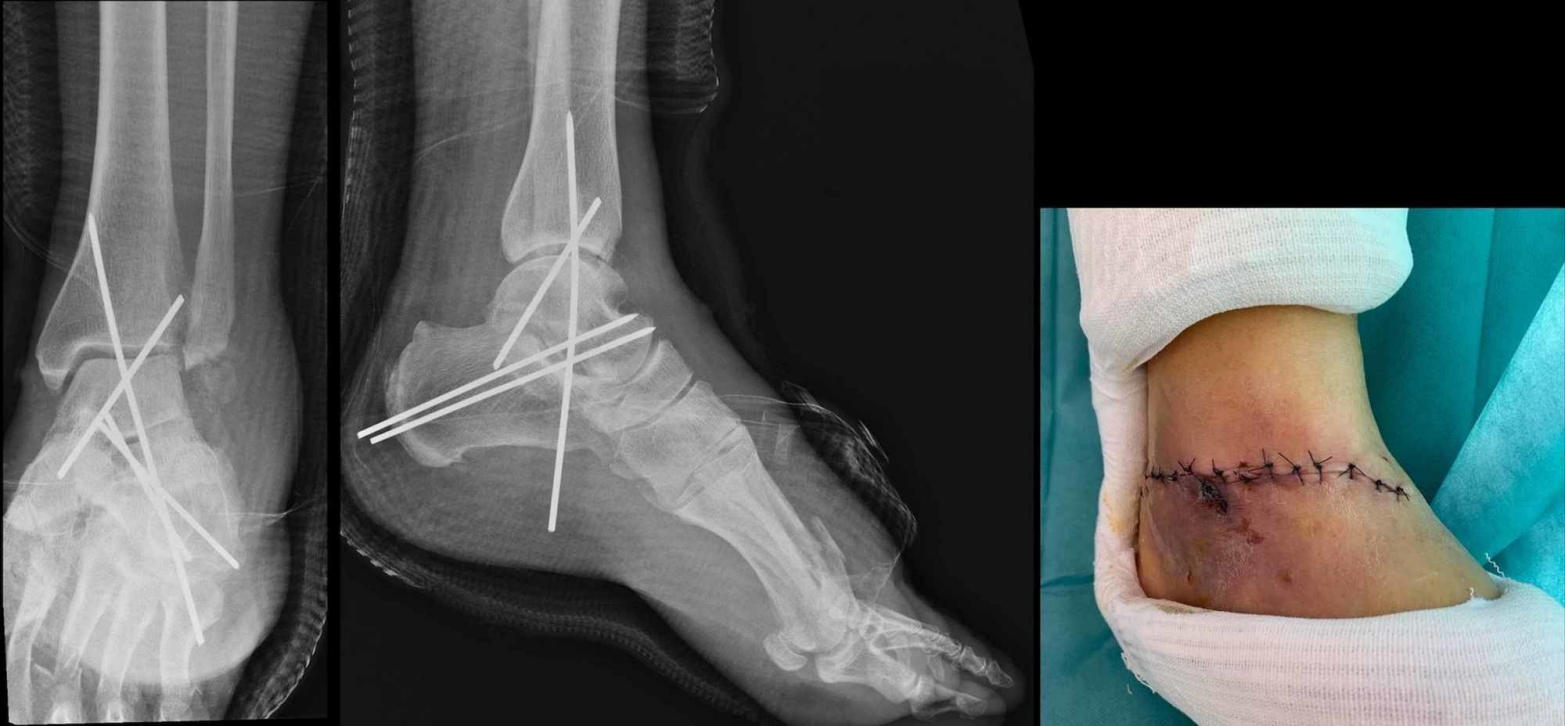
Postoperative radiographs showing a good reduction of the talus and fixation with 4 K-wire (22/10) through the talocrural and subtalar joints
